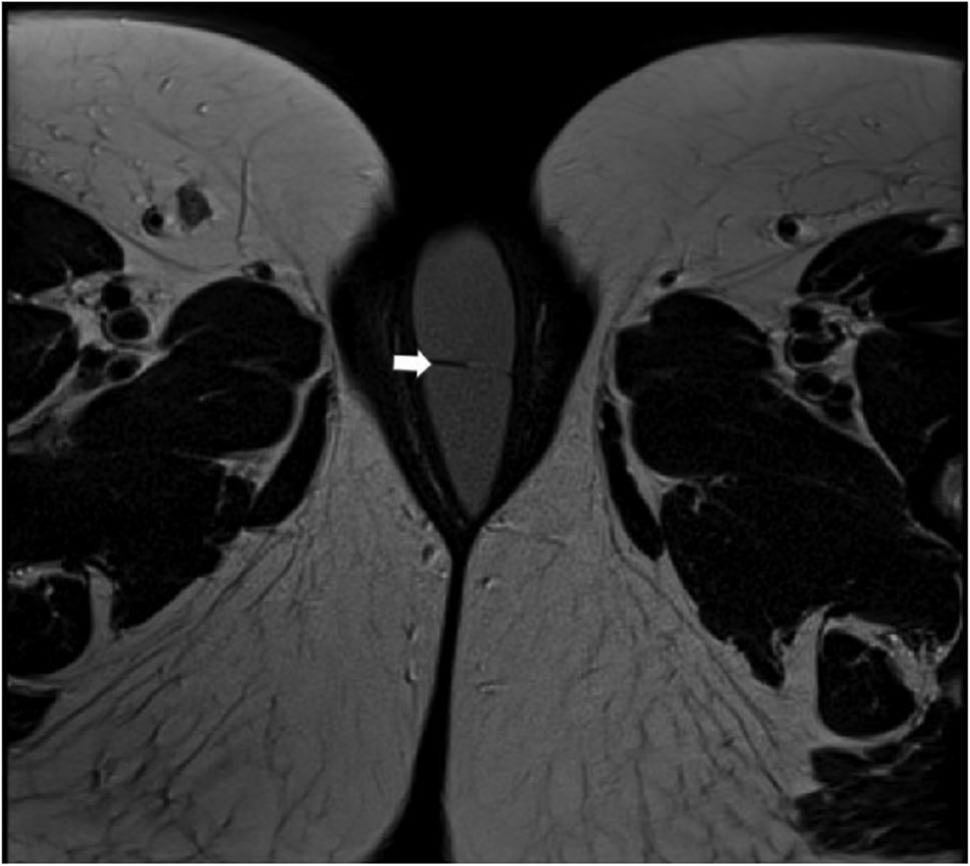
MRI transverse view of clitoral cyst
